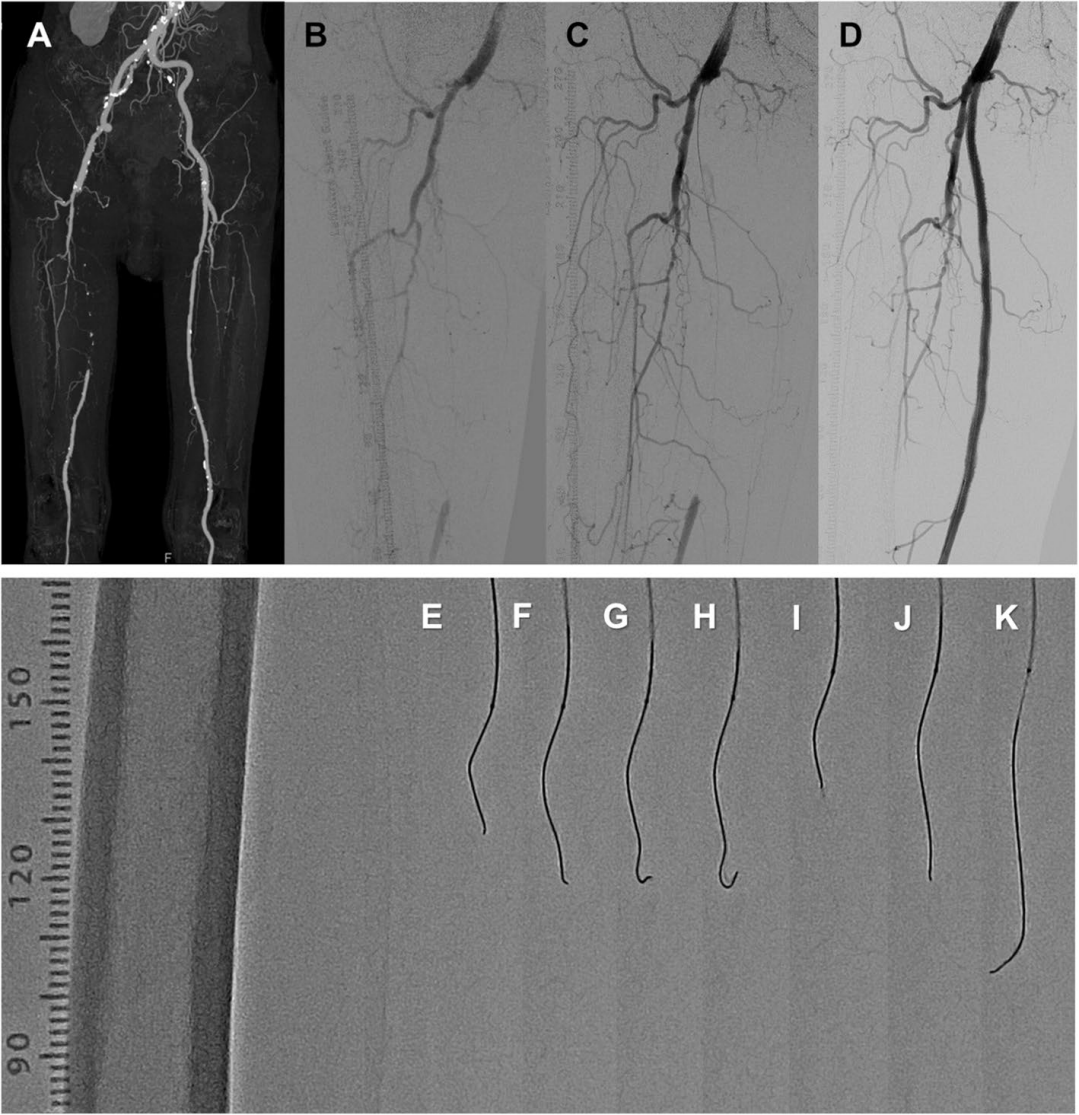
A representative case of GLIMGLIN. a A computed tomographic angiography revealed a right SFA CTO. Angiography of pre-EVT (b), after the wire crossing (c), and post EVT (d). e-k Shapes of a 0.018-inch Gladius MG™ during GLIMGLIN. When the first point was bending (g, h), the wire pulled back (i). The wire was rotated to select a different route for its advancement without bending at the first and second points (j, k)
